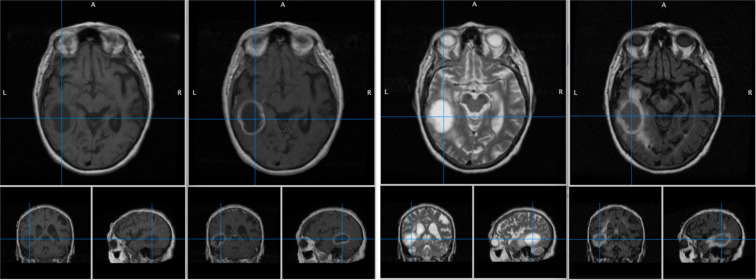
An example of four modalities (T1-weighted, T2-weighted, post-contrast T1-weighted (T1-C), and FLAIR) from the same brain cancer patient (patient# HF1702).
